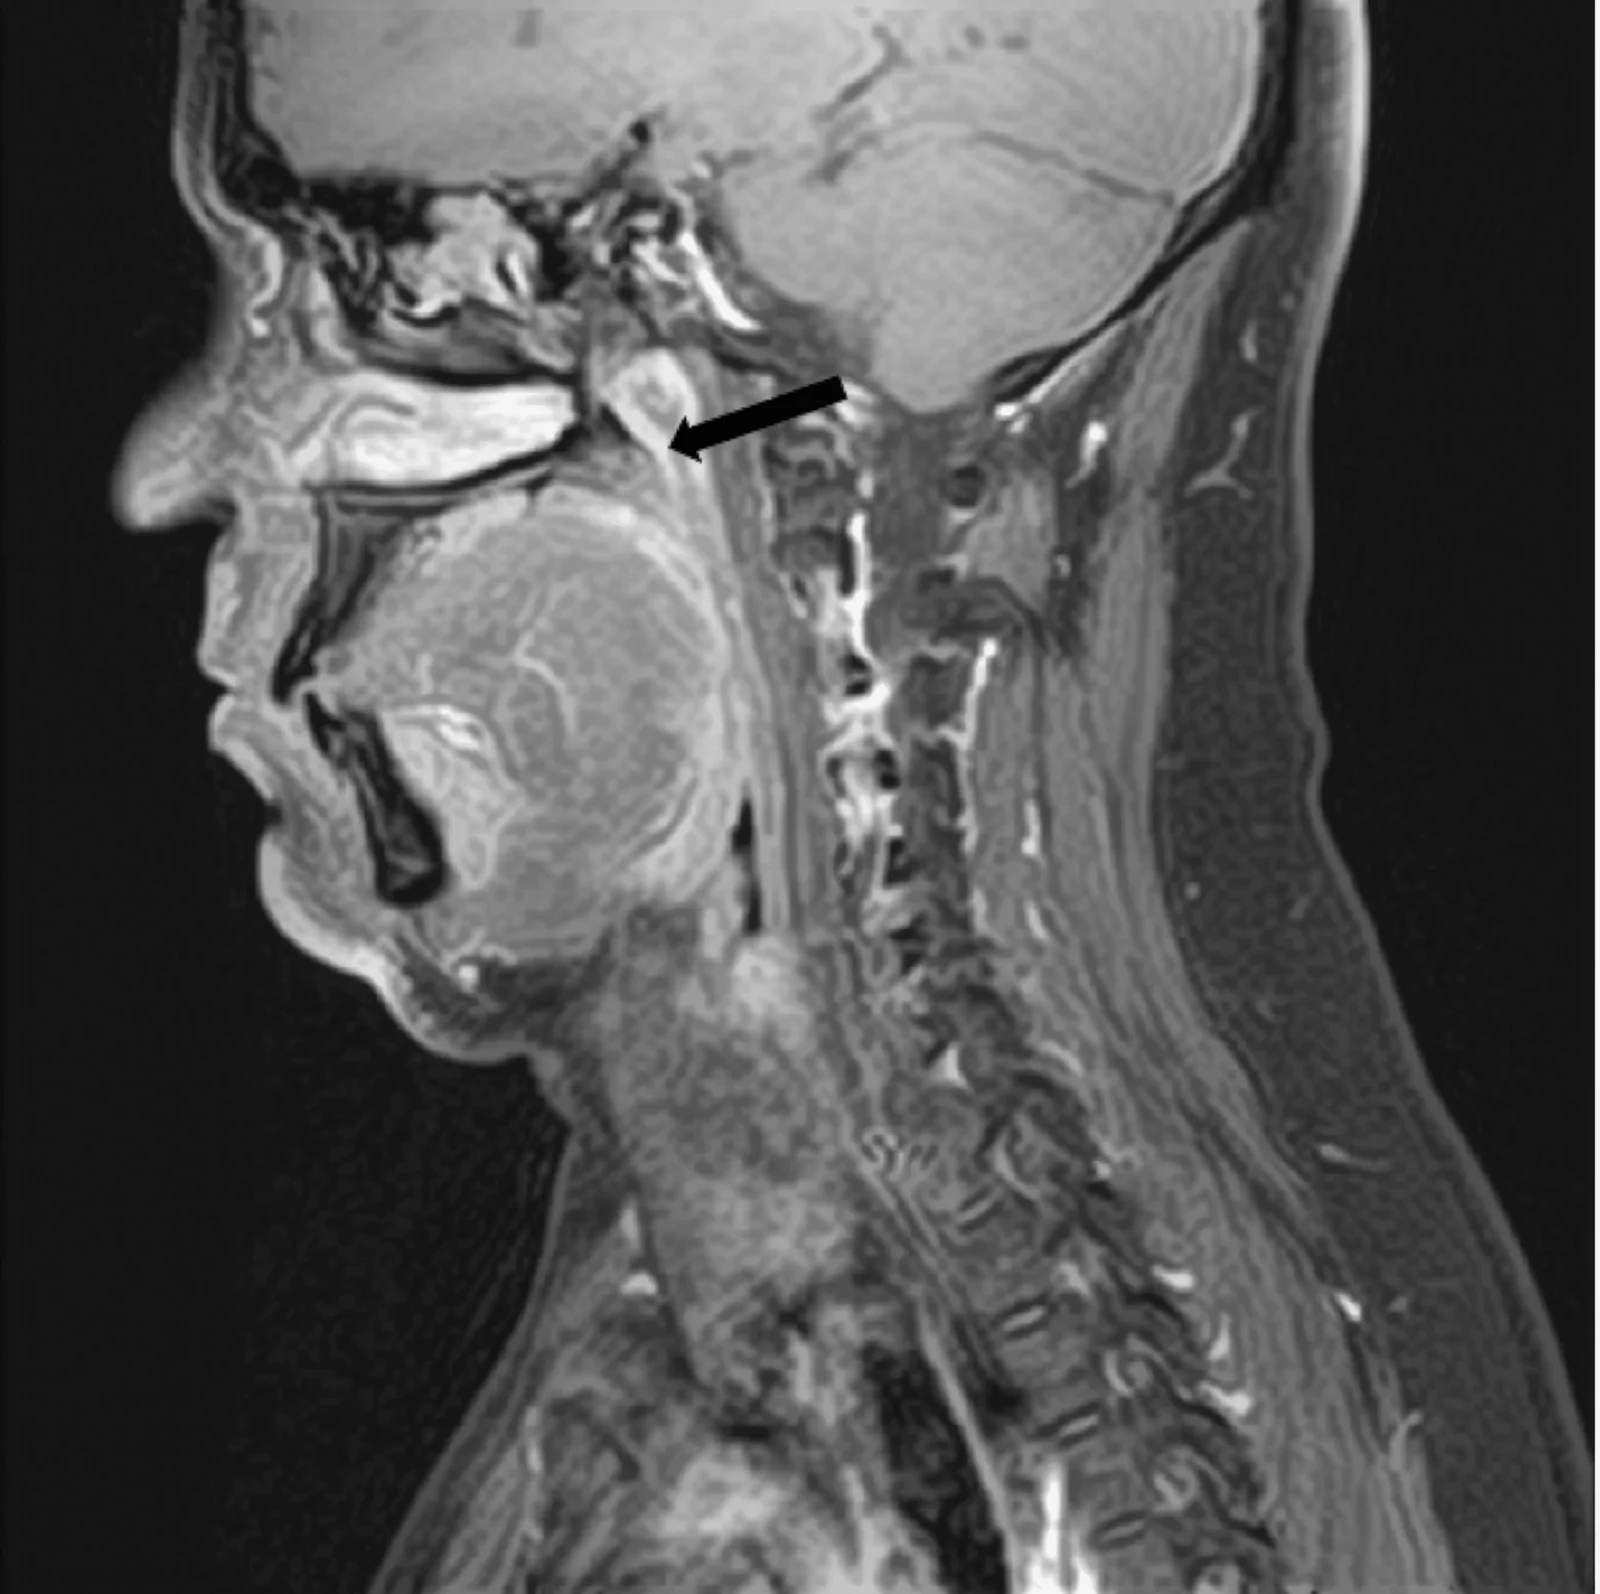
Magnetic resonance imaging findings. T1 contrast-enhanced sagittal MRI of the face demonstrating the right hard palate lesion.
